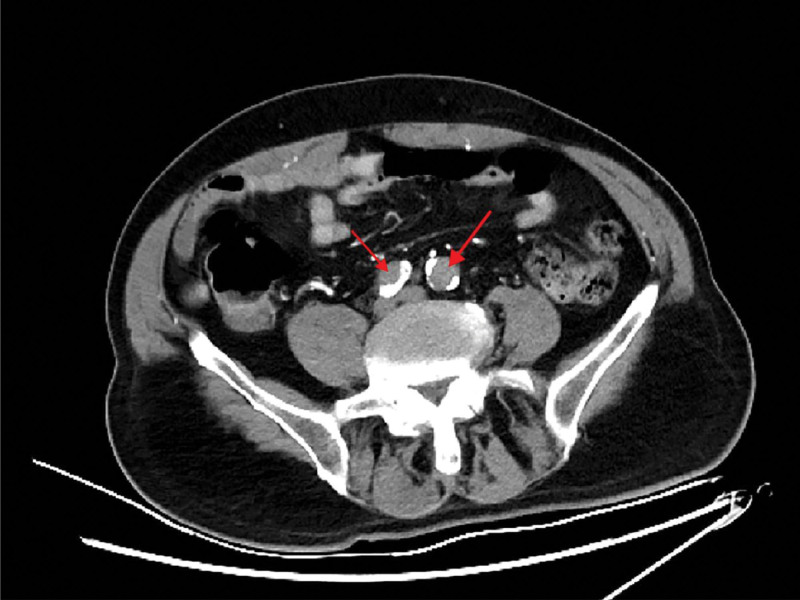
Computed tomography angiography of the chest and abdomen revealed bilateral occlusion of the common iliac arteries.
